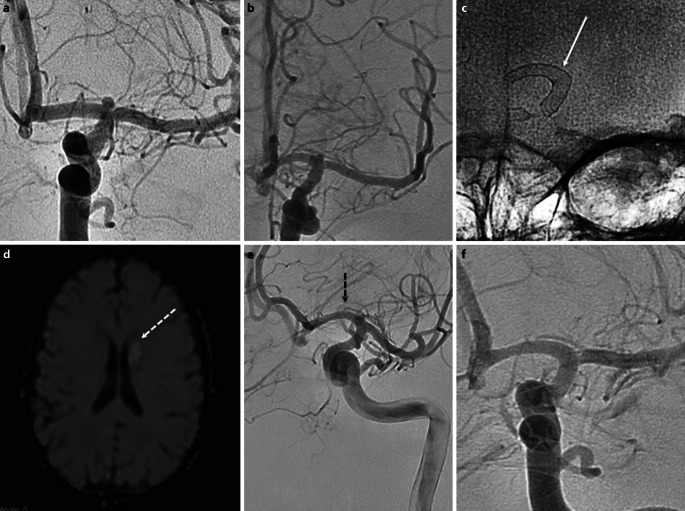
An incidental carotid bifurcation aneurysm (a) was found in a patient (patient no. 19) undergoing investigation for headache. After discussion with the patient endovascular treatment for the aneurysm was performed that involved deployment of a single p48MW 3.0 × 18 mm from the A1 and back into the terminal ICA crossing the aneurysm origin (b, c, *white arrow*). There were no intraoperative complications; however, the patient was found to have unilateral weakness postoperatively. An urgent MRI revealed diffusion restriction within the caudate head (d, *dashed white arrow*) suggestive of infarction within the territory of the artery of Heubner. A repeat catheter angiogram was performed which revealed patency of the FDS and the Heubner artery (e, *dashed black arrow*). The patient made a complete recovery and the mRS was 0 at 90 days and at last clinical follow-up (12 months). The aneurysm was completely excluded from the circulation at follow-up angiography (12 months) (f)
